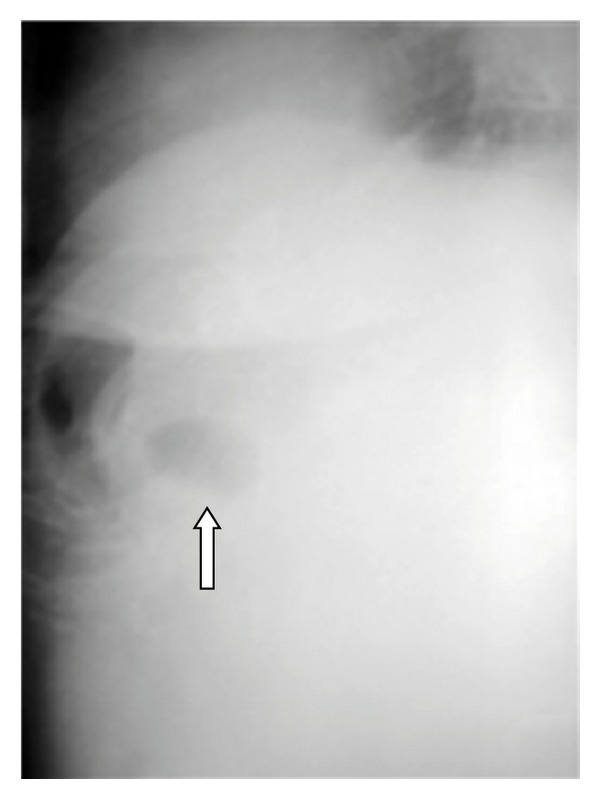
A plain abdominal X-ray suspecting the presence of air in the gallbladder (arrow).
